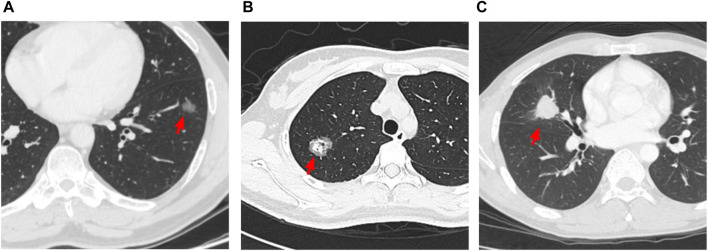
Representative images of 3 different characteristics of CT. (A) red arrow: pure GGO nodule with diameter of 1.6 cm; (B) red arrow: part GGO nodule with diameter of 2.6 cm; (C) red arrow: pure solid nodule with diameter of 2.6 cm.
